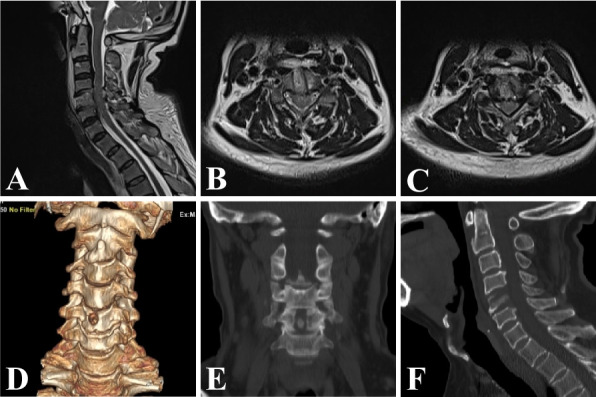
One week after surgery, sagittal and axial MRI images showed significant decompression (A-C). The cervical CT-3D reconstruction and coronal images showed the clear position of the entrance and two bony channels (D, E), and the CT sagittal image showed that the grafted autologous bone was complete and most of the osteophyte was removed (F)
